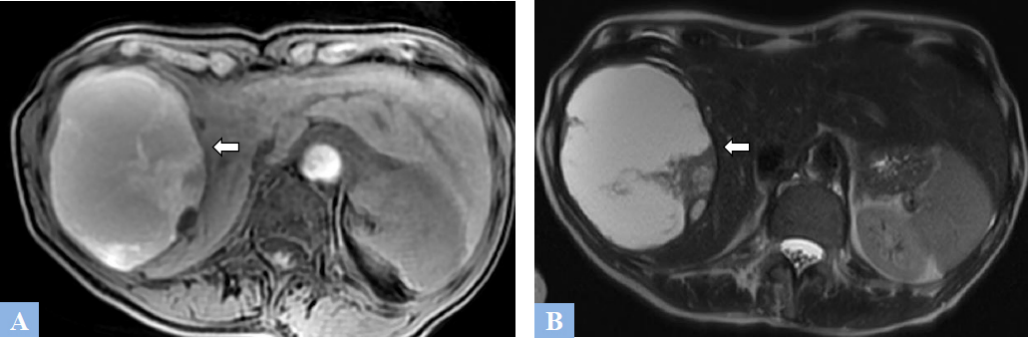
Hemorrhagic biliary cyst in a 76-year-old female patient presenting with right hypochondrium pain. MRI features. A. T1-weighted fat-suppressed MR image in the axial plane. Cystic lesion in the right liver with spontaneous T1 hyperintensity, consistent with hemorrhage. B. T2-weighted fat-suppressed image in the axial plane showing heterogeneous content of the hepatic cyst, consistent with hemorrhage.
